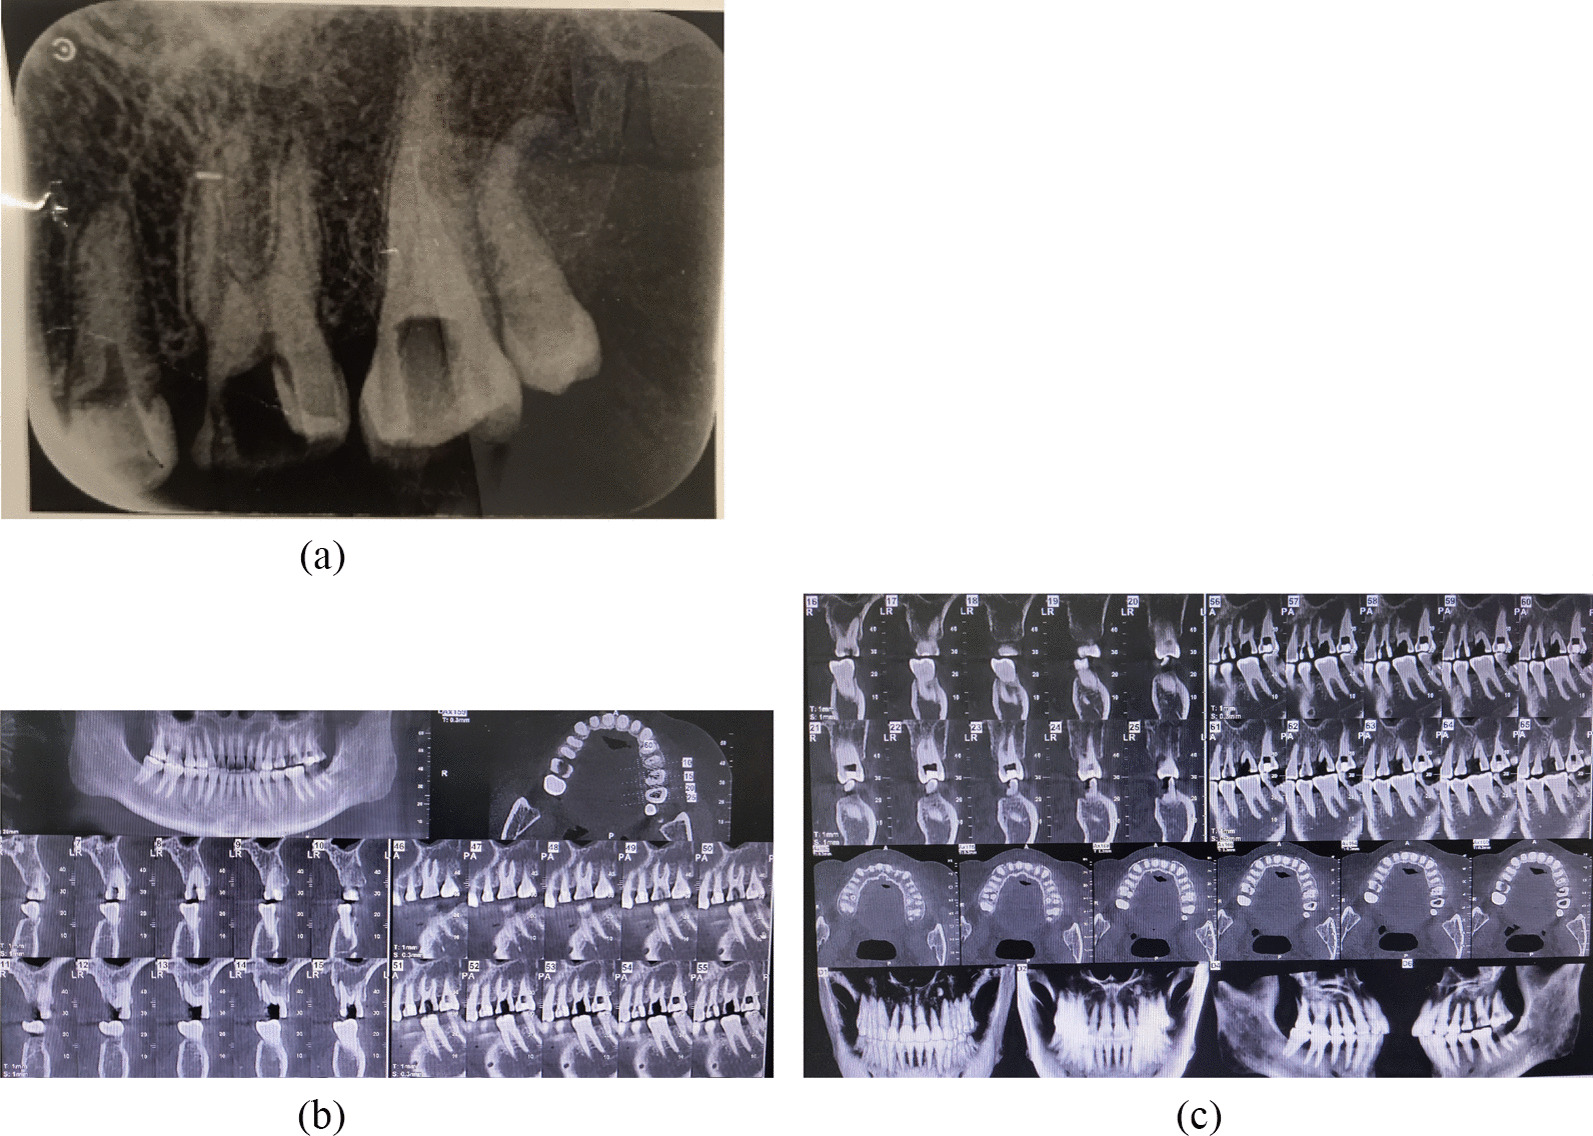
Preoperative dental and CBCT radiographs show generalized pulpal obliteration. The pulp chamber is completely absent in almost all maxillary and mandibular teeth
